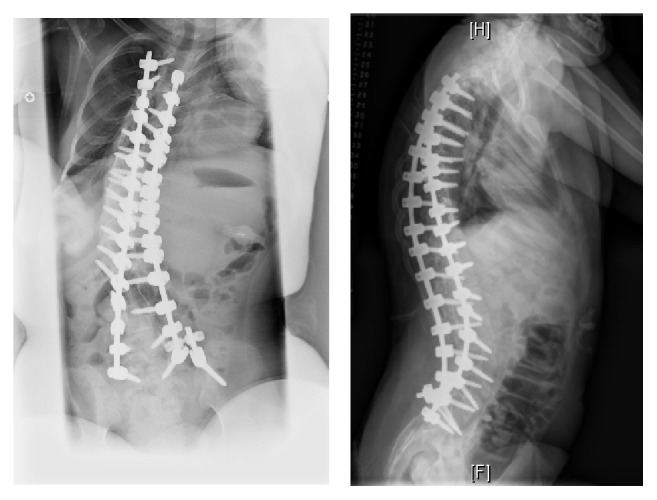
Immediate postoperative radiograph images showing spinal fusion and instrumentation from T3-S1 with significant improvement of the deformity. Unilateral pelvic fixation was carried out in this patient.
